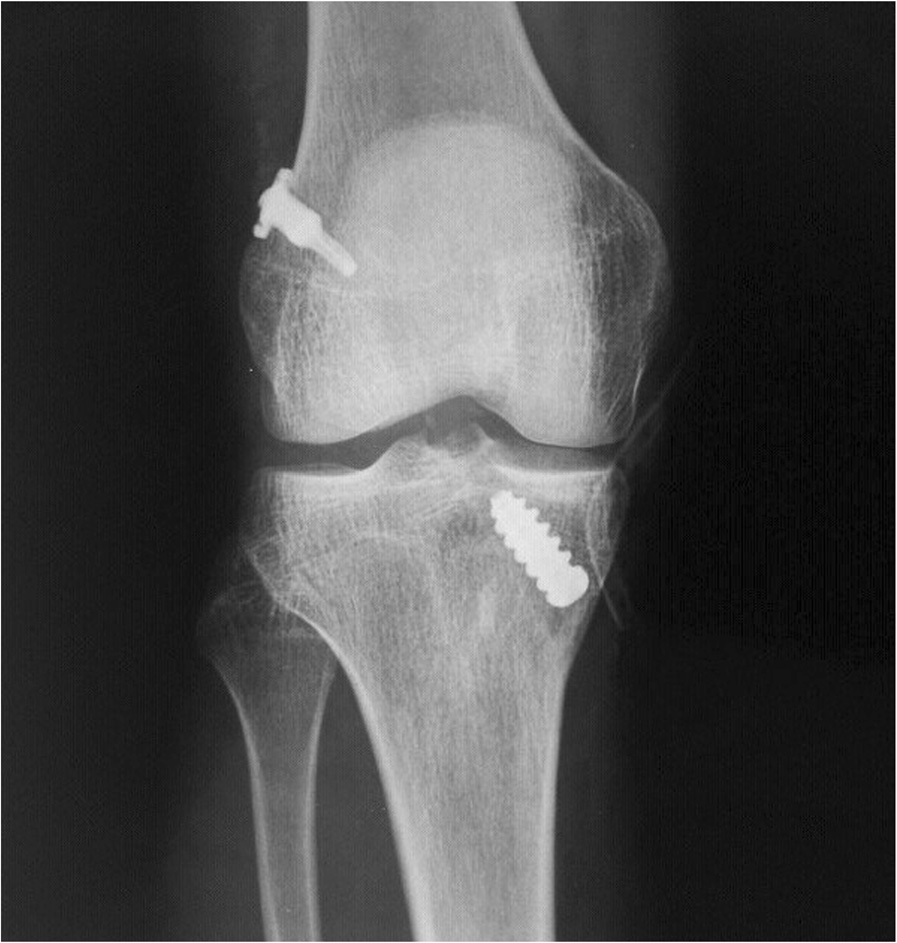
Postoperative anterior-posterior radiograph of an anterior cruciate ligament reconstruction, showing femoral fixation with the ETD®.
